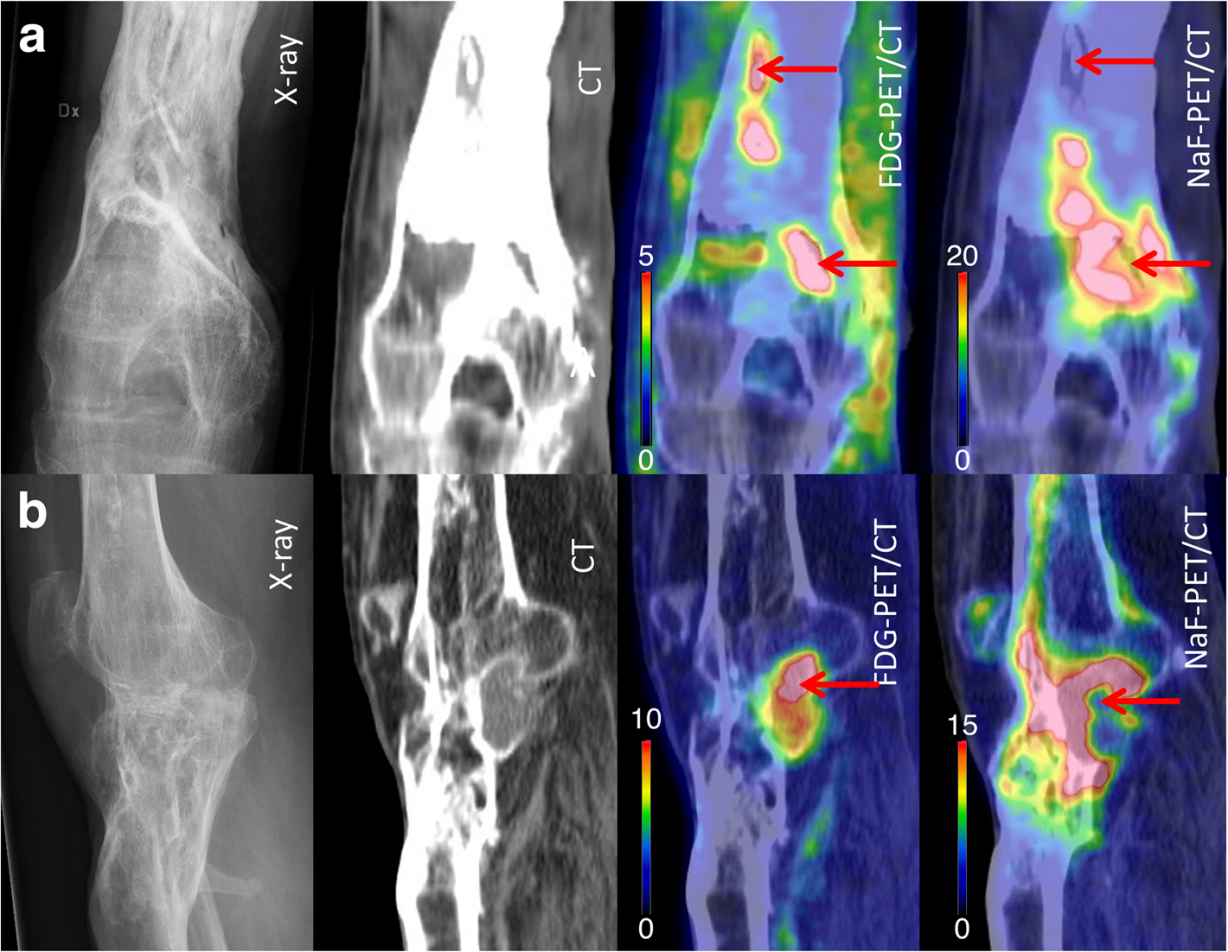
Preoperative images (X-ray, CT, FDG-PET/CT, and NaF-PET/CT) of chronic osteomyelitis in distal femur in two different patients. The arrows point at bone segments with high uptake on FDG-PET/CT (“hot spots”) and low uptake on NaF-PET/CT (“cold spots”). The findings are best visualized in coronal views in patient (a), where two sequestra were found, and in sagital views in patient (b), where one sequestrum was found
